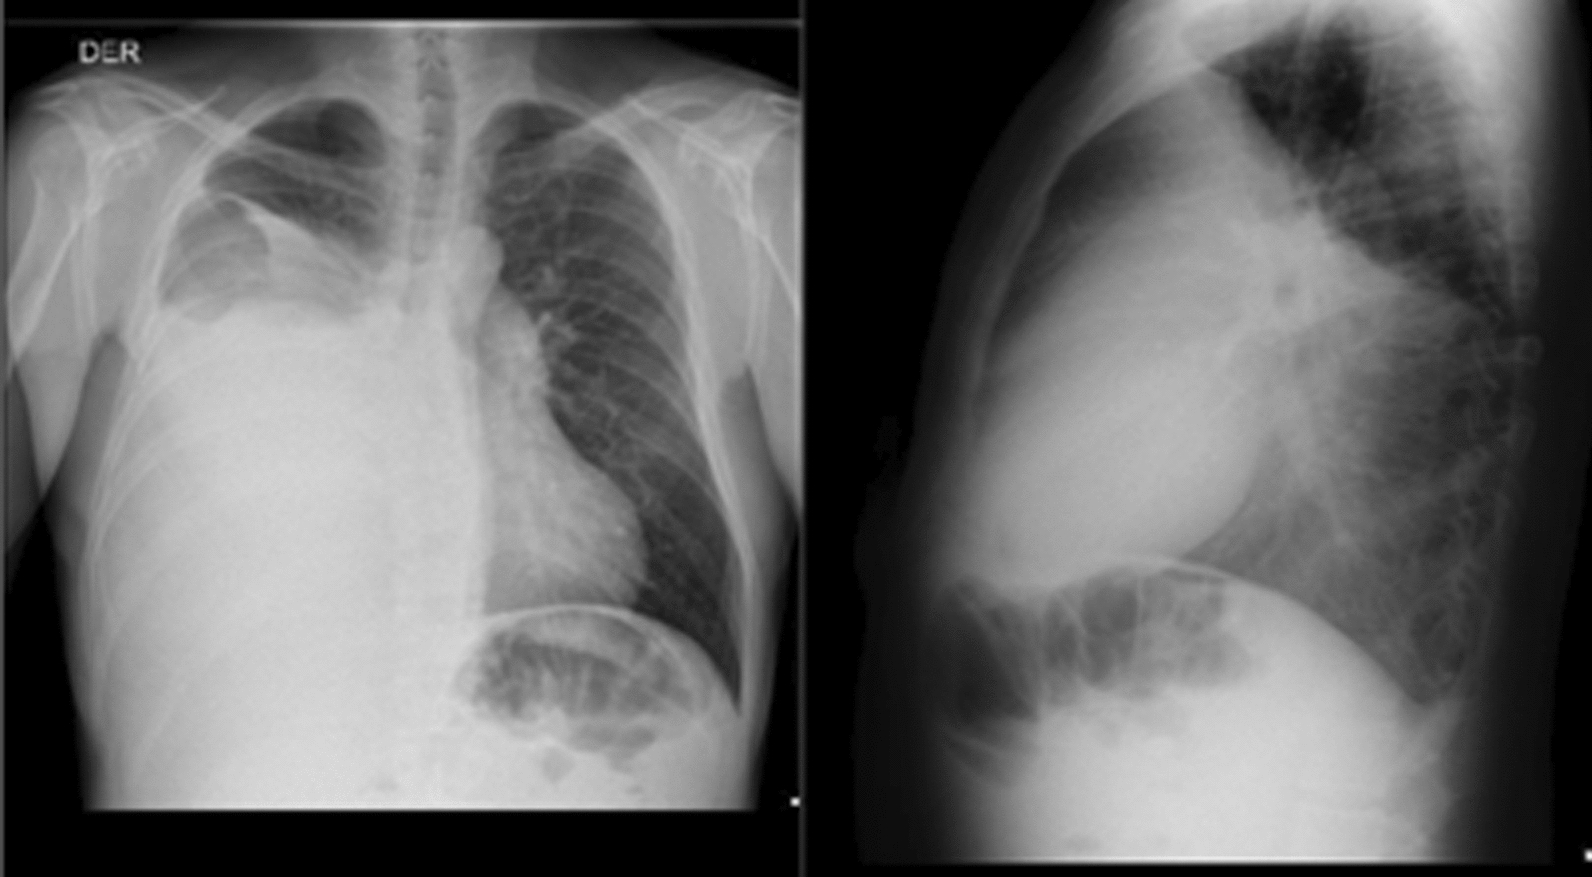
Anteroposterior (AP) and lateral chest radiographs, highlighting a radiodense space-occupying lesion with ill-defined borders and mild ipsilateral pleural effusion, accompanied by subsegmental atelectasis in the ipsilateral hilar regions
